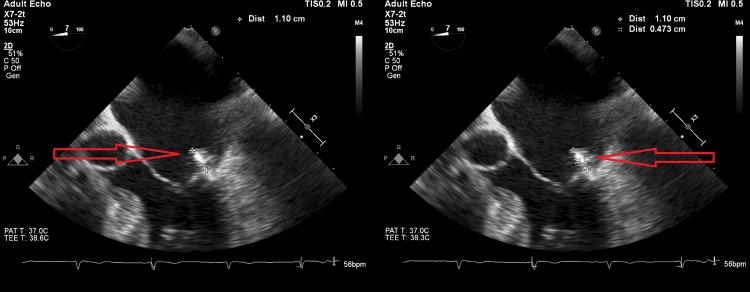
TEE demonstrating a pedunculated 1.0 × 0.7 cm mass on the annulus of the anterior leaflet of the MV, which appears to be connected by a stalk. MV: Mitral valve; TEE: Transesophageal echocardiogram.
